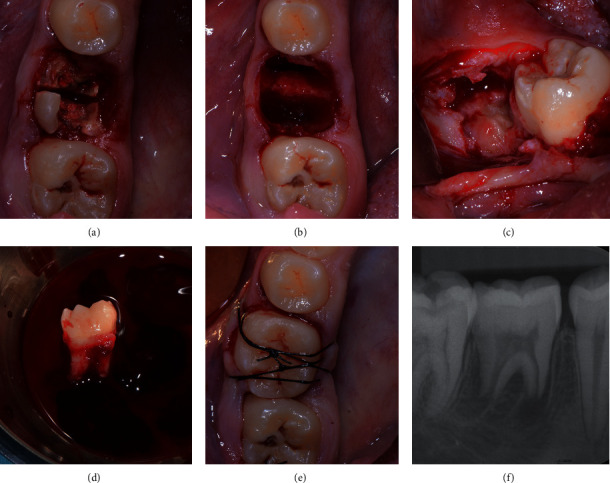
Tooth 46: (a) root's separation; (b) alveolar fresh extraction socket; (c) impacted right third molar; (d) third molar donor tooth extracted and stored in the L-PRF exudate; (e) transplanted tooth in position 46 and cross-sutures for stabilization; (f) periapical radiograph immediately after transplantation.
